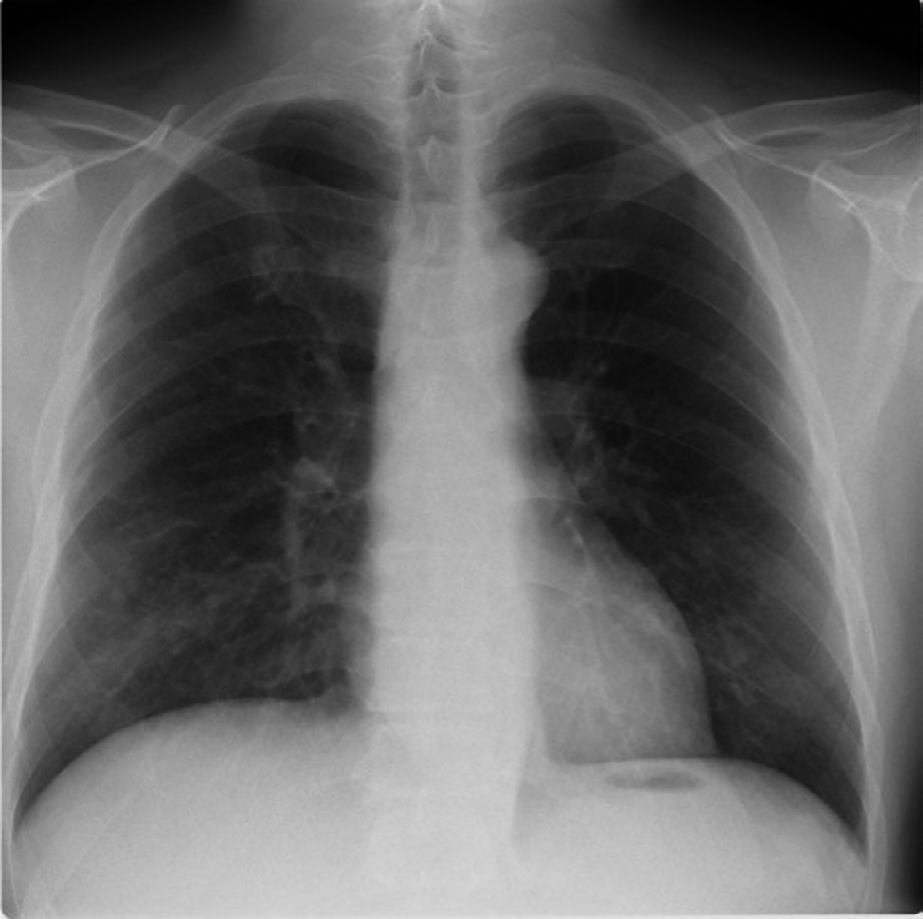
A chest radiograph only showing bilateral ground‐glass shadows
